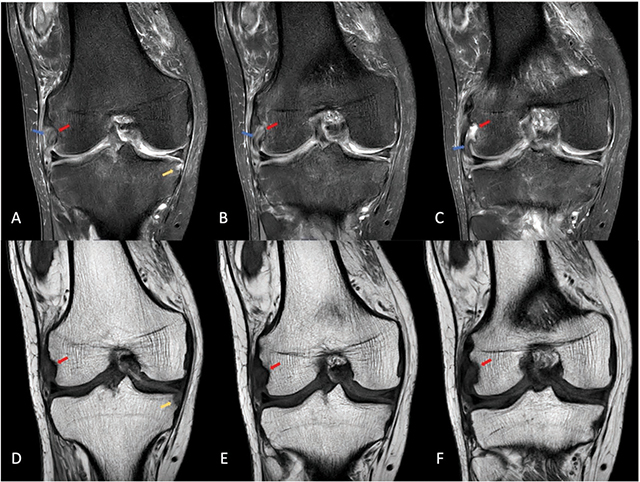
MRI of the right knee demonstrating a diffusely thickened, hyperintense popliteal tendon on proton density-weighted images (blue arrows), an erosion of the popliteal sulcus (red arrows) and an erosion at the medial rim of the medial tibial plateau (yellow arrow).
